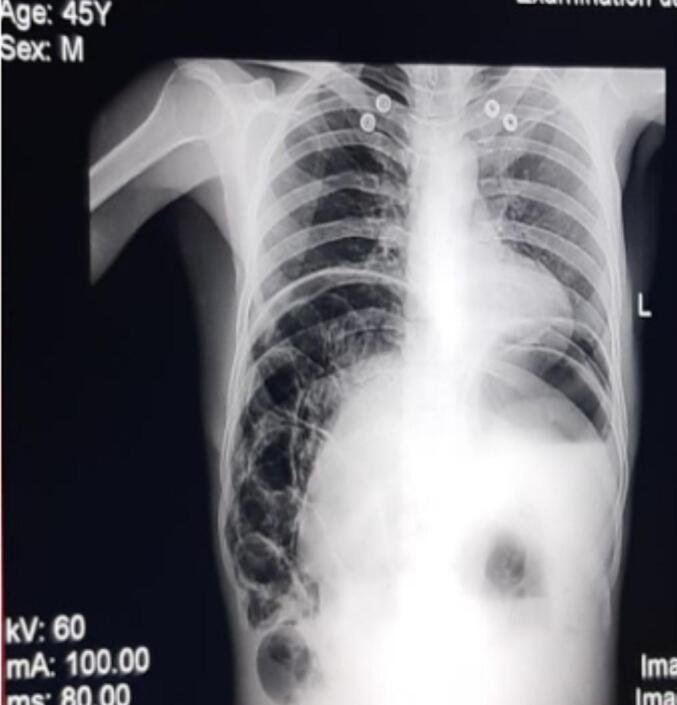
An abdominal x-ray shows free air under the right hemidiaphragm (blue arrow) and multiple cystic radiolucent areas within the bowel wall (white arrow).
